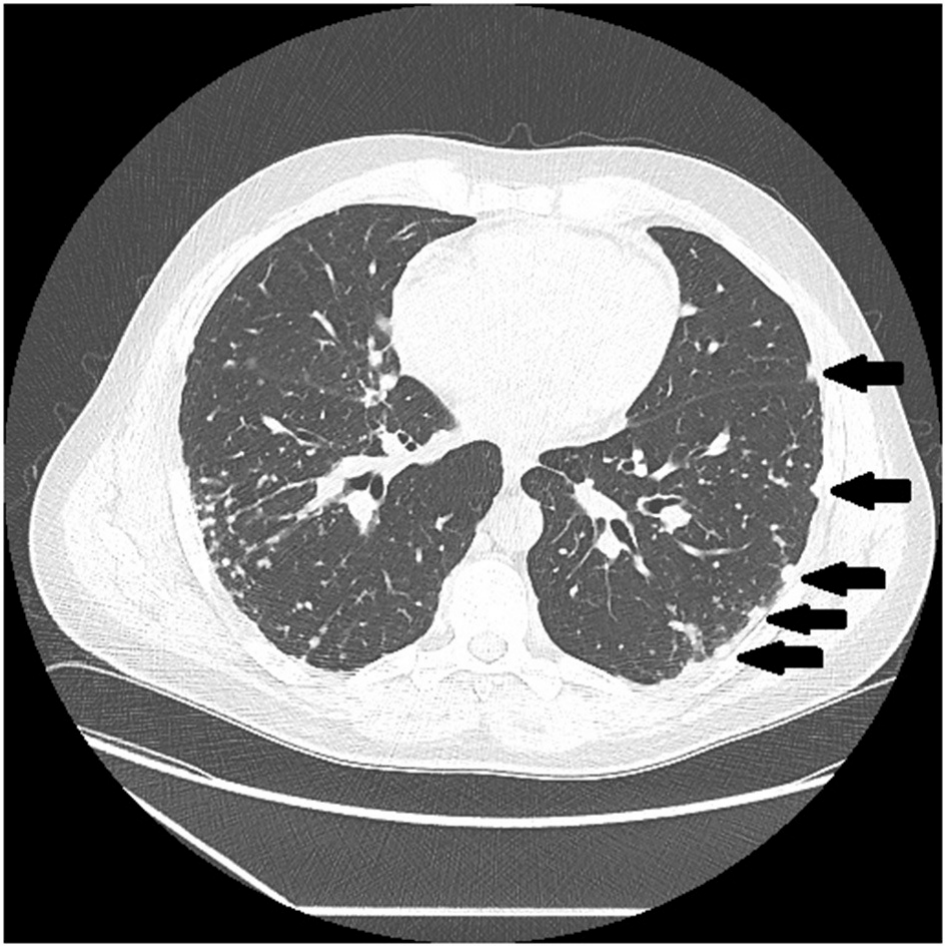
Chest CT scan showing multiple subpleural nodules from sarcoidosis (arrows).
